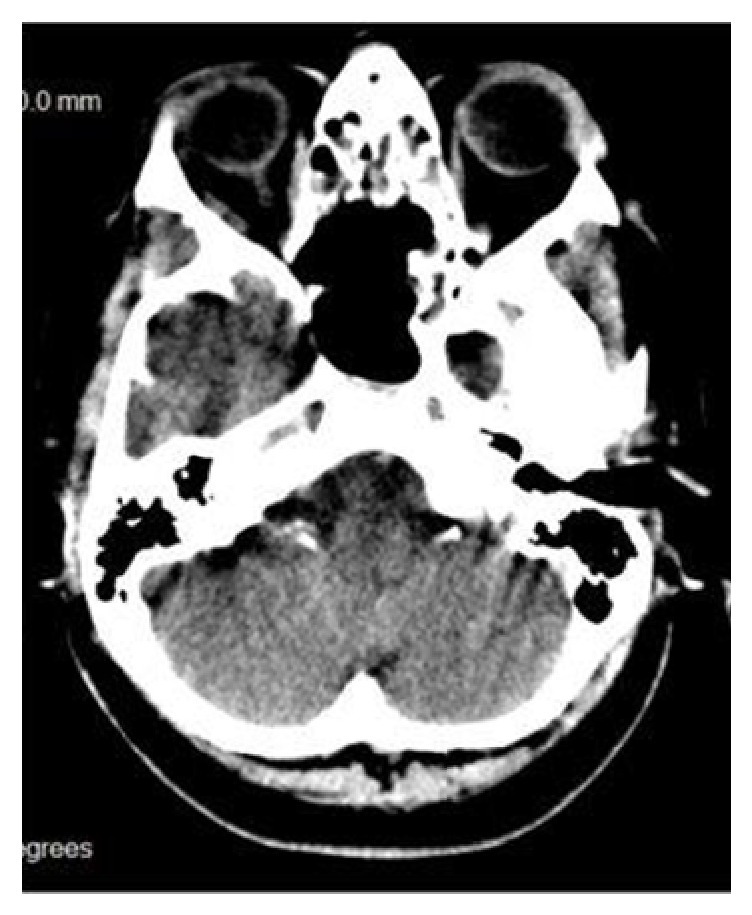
Postoperative CT scan showing the sphenoidotomy, the posterior ethmoidectomy, and the absence of lesion.
